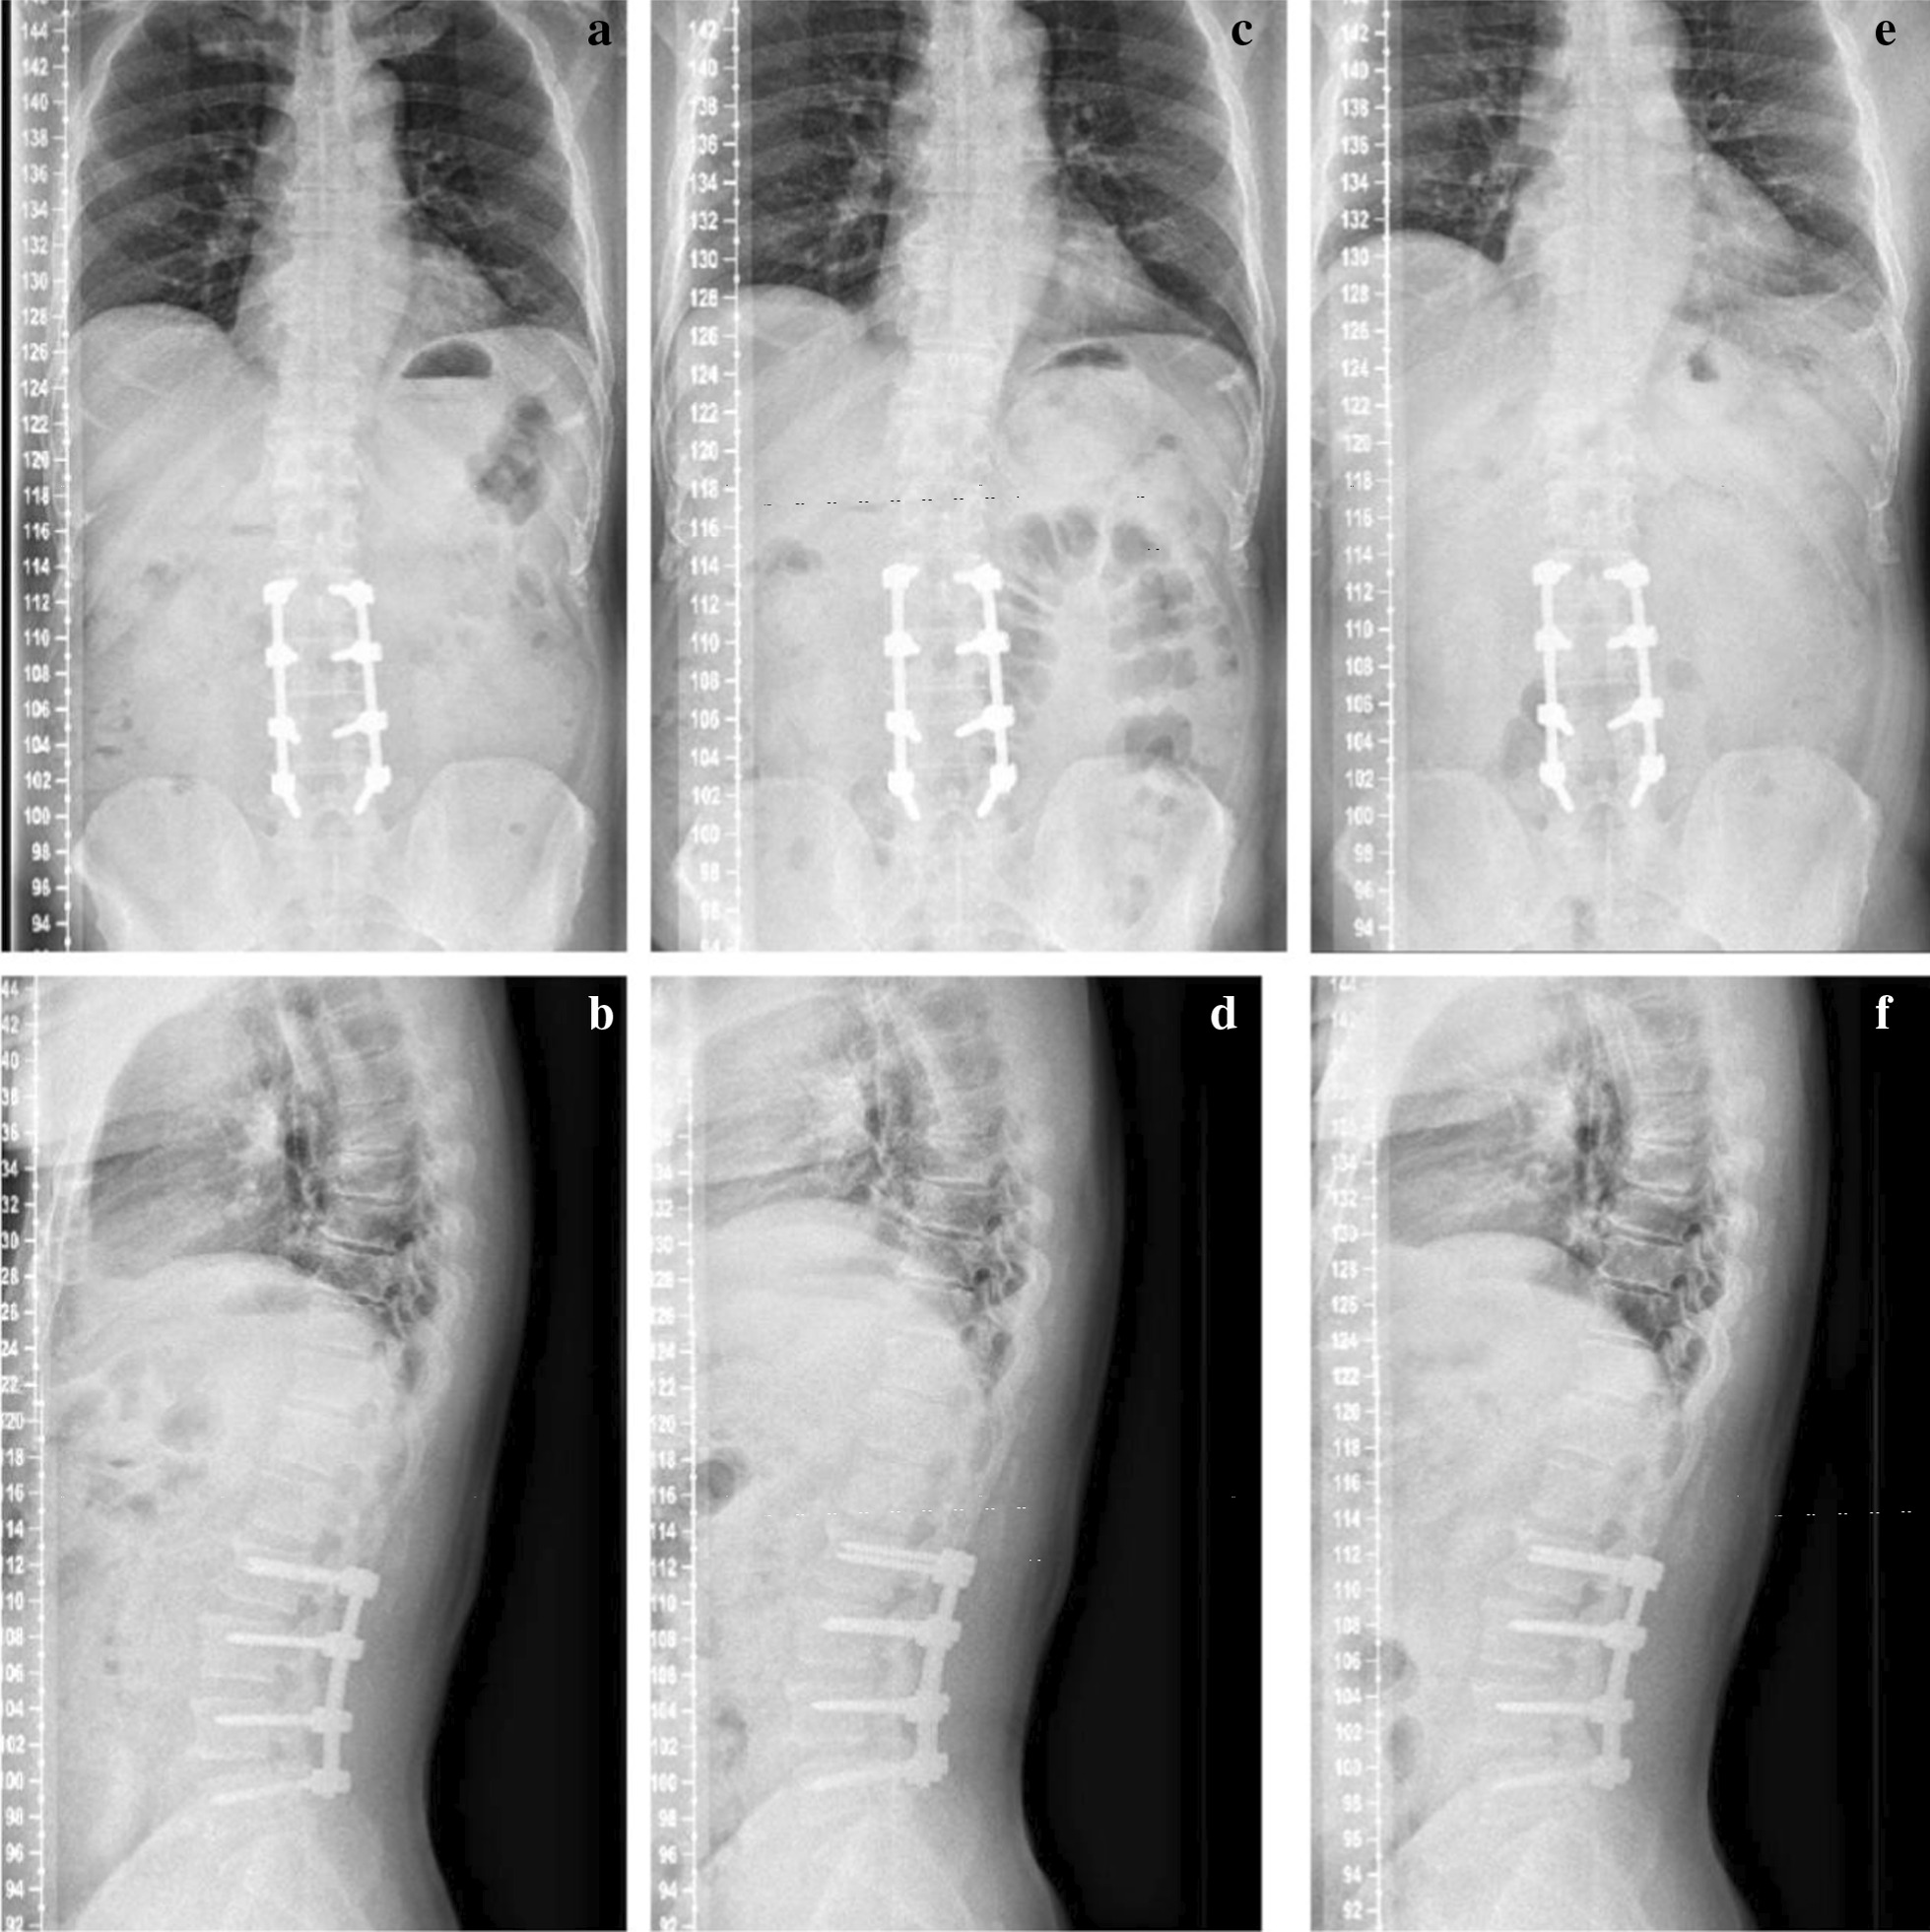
Follow-up X-Rays. Follow-up imaging examination shows consistent spinal stability and excellent internal fixation. a Coronal image of 3 months after surgery. b Sagittal image of 3 months after surgery. c Coronal image of 6 months after surgery. d Sagittal image of 6 months after surgery. e Coronal image of 22 months after surgery. f Sagittal image of 22 months after surgery
